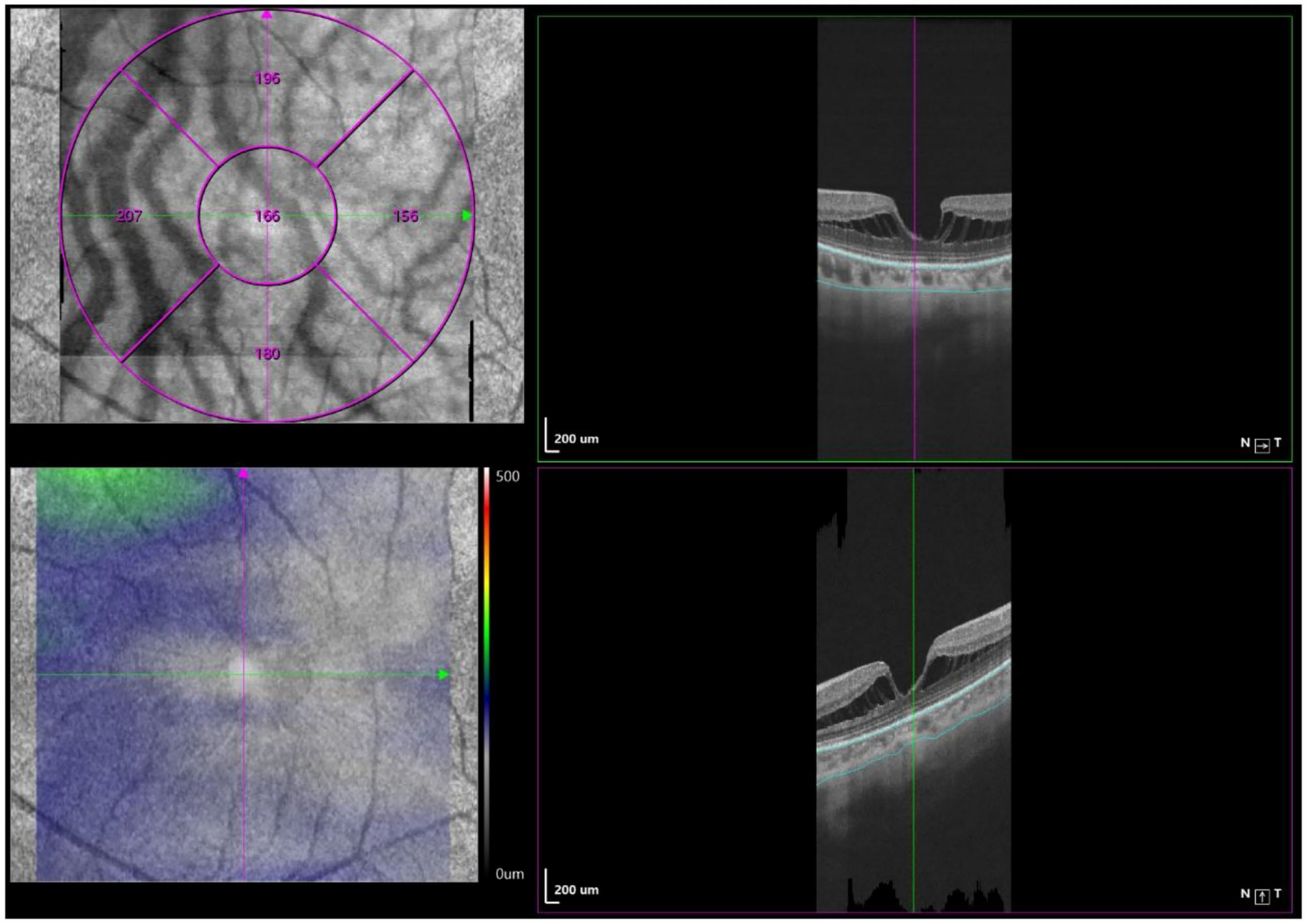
Macular CT measurement by swept-source OCT angiography. Macular CT was separately calculated in 5 regions (fovea, tempo, superior, nasal, and inferior) based on ETDRS contour. The choroid was defined as the volume from the basal border of the RPE–Bruch's membrane complex to the chorioscleral junction.
